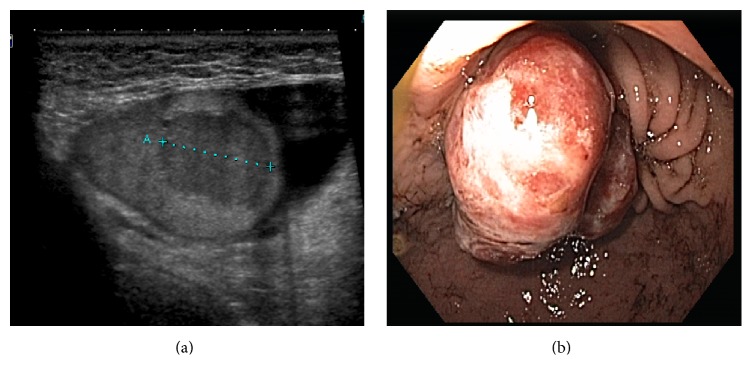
(a) Ultrasound showing testicular metastasis of RCC; mass measuring 2.1 × 1.7 × 2.0 cm. (b) Endoscopy showing metastatic RCC mass to the fundus of the stomach.
